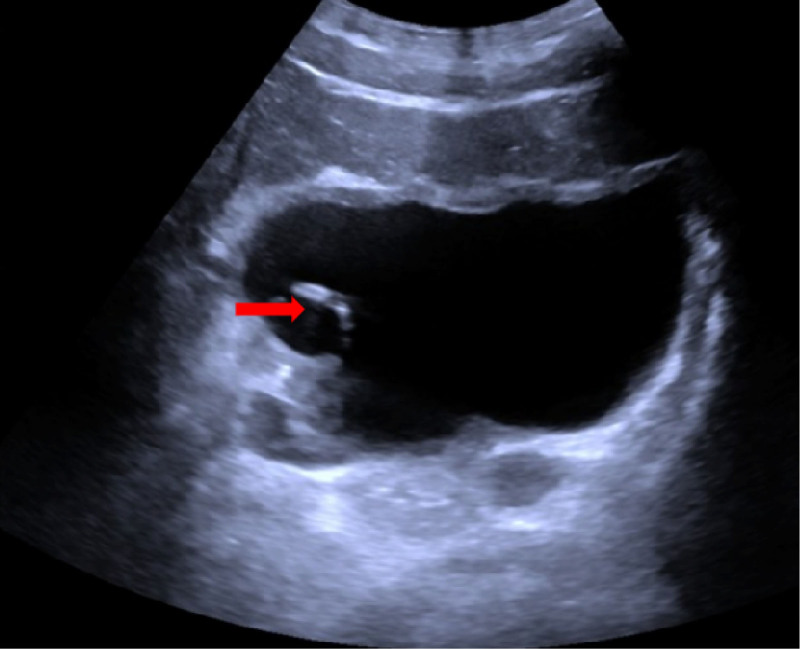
Ultrasound demonstrated a “J” stent in the right ureter and bladder, with no evidence of hematoma around the kidney or ureter.
